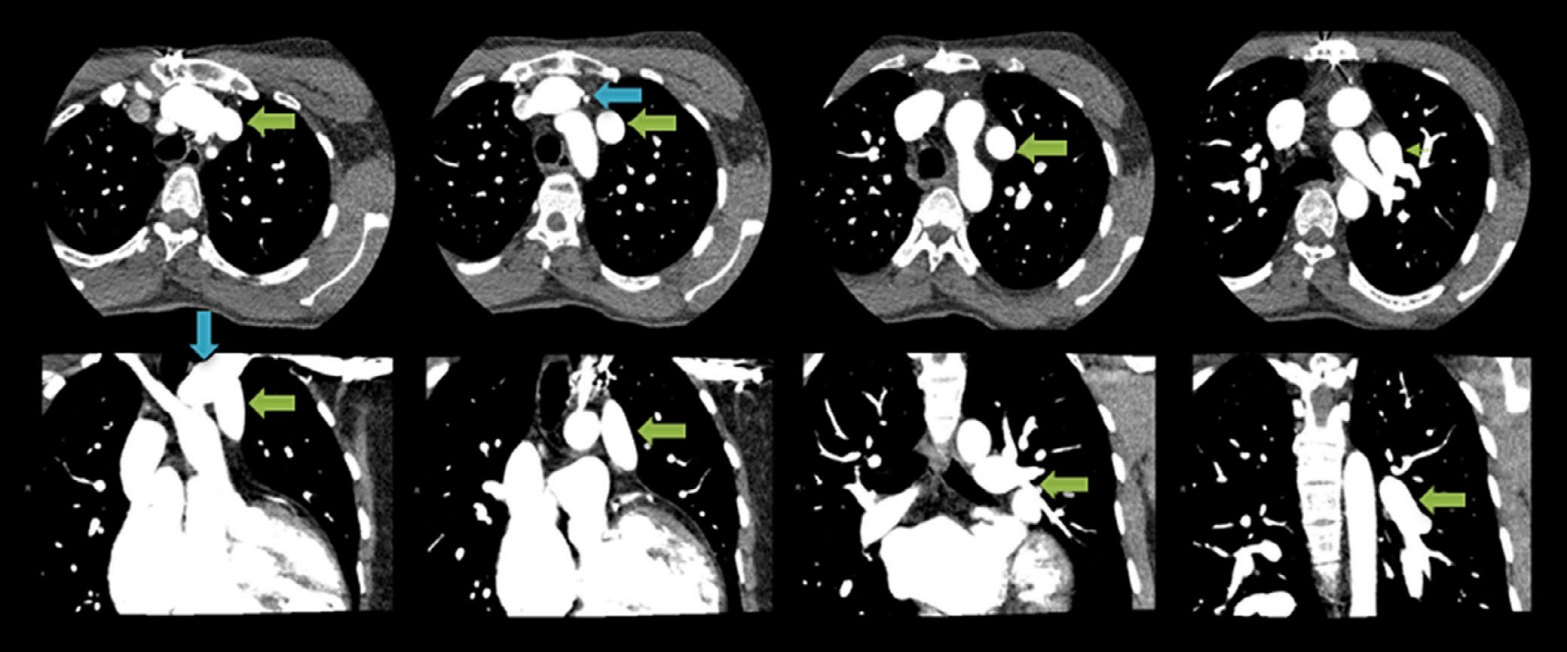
Preoperative CT‐angiogram scan in coronal (bottom panel) and transversal plan (top panel), demonstrated left pulmonary vein (PAPVR) (green arrows) draining into left brachiocephalic vein (blue arrow)
